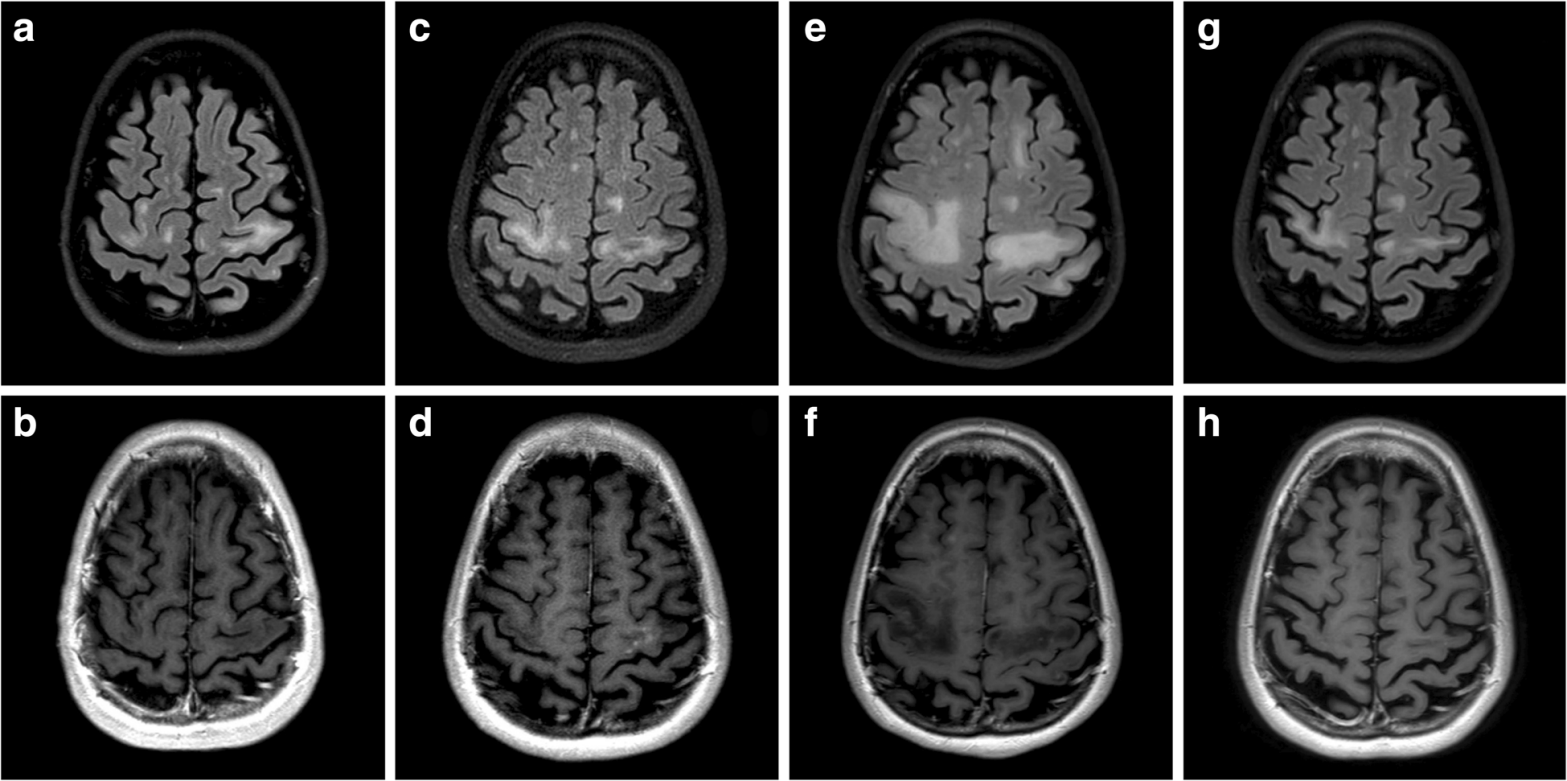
(a-h) Magnetic resonance imaging T2 fluid-attenuated inversion recovery and post-contrast T1 images of the patient at time of progressive multifocal leukoencephalopathy diagnosis showing bilateral asymmetric confluent non-enhancing hyperintensities in the subcortical precentral gyri (a, b), at 3 months showing a few small enhancing lesions in the left frontal lobe suggestive of immune reconstitution inflammatory syndrome (c, d), at 5 months showing interval development of T2 signal abnormality with mild enhancement (e, f), and at 1 year showing further decrease in T2 hyperintensities and resolution of enhancement (g, h)
